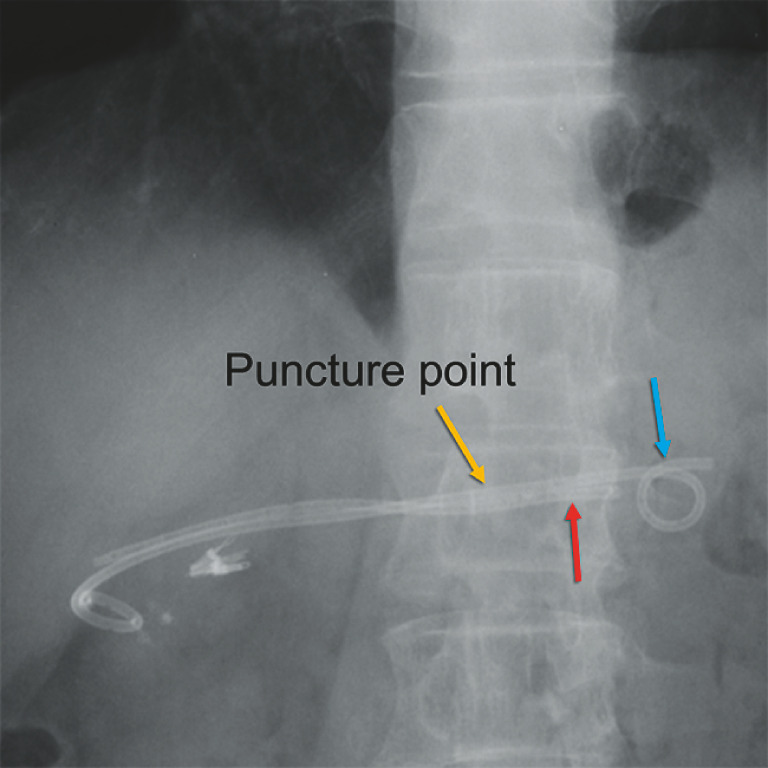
An X-ray image of this case. Yellow arrow: puncture point. Red arrow: gastric end of a straight plastic stent that had migrated to the pancreatic tail side. Blue arrow: gastric end of a double-pigtail plastic stent in the stomach.
